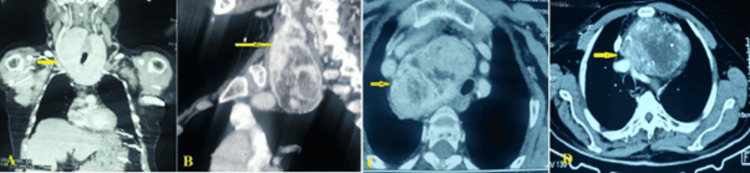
CT scan showing retrosternal thyroid mass (A) coronal view (arrow), (B) lateral view (arrow), (C) mass causing tracheal deviation (arrow), and (D) big thyroid mass with calcifications (arrow) CT: computed tomography
